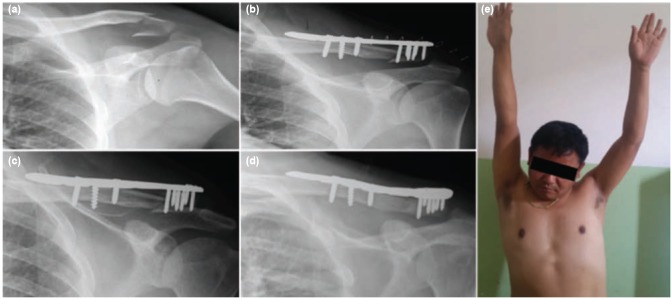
(a) Antero-posterior radiograph of shoulder joint showing comminuted lateral third clavicle fractures, (b) Immediate postoperative radiograph after fixation with pre-contoured lateral third clavicular plate, (c) Radiograph of clavicle 6 weeks after surgery, (d) Radiograph of clavicle 20 degrees caudal AP view 5 months after surgery showing bony union and (e) Abduction of shoulder joint 6 weeks after surgery.
